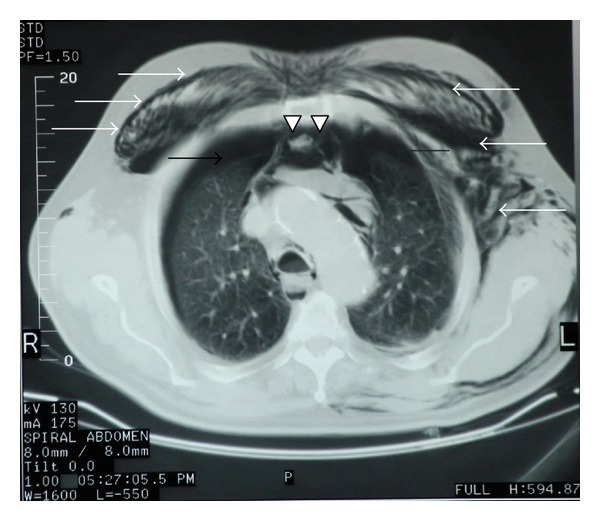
Computed tomography scan of the thorax. Bilateral pneumothorax (black arrows), pneumomediastinum (arrow heads) and subcutaneous emphysema of the anterior and lateral thoracic wall (white arrows).
